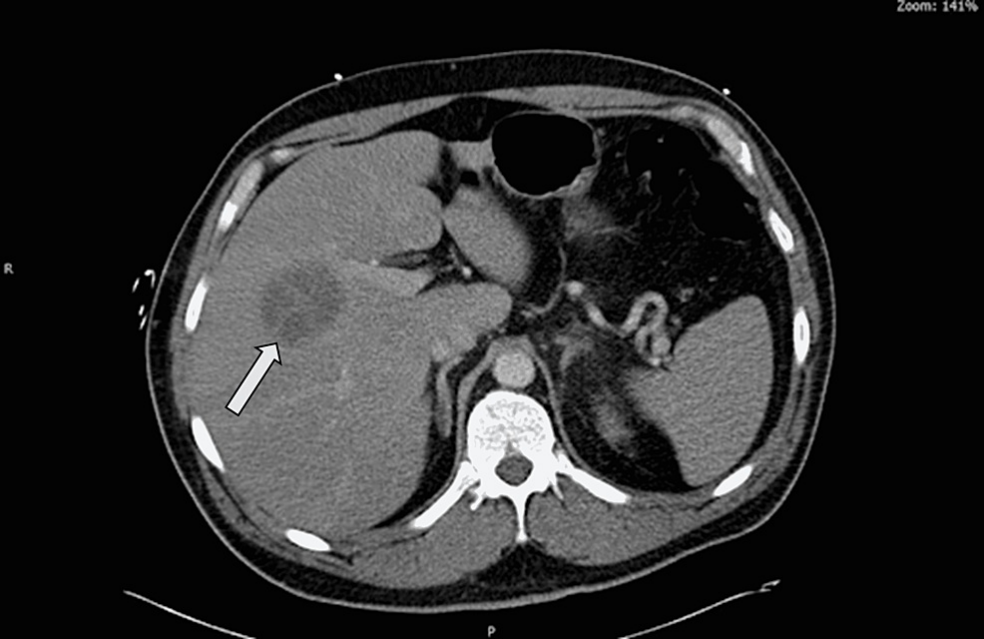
Computed tomography (CT) scan of abdomen and pelvis showing a septated hypodense lesion of 4.8 x 4.5 cm in central liver (arrow).
